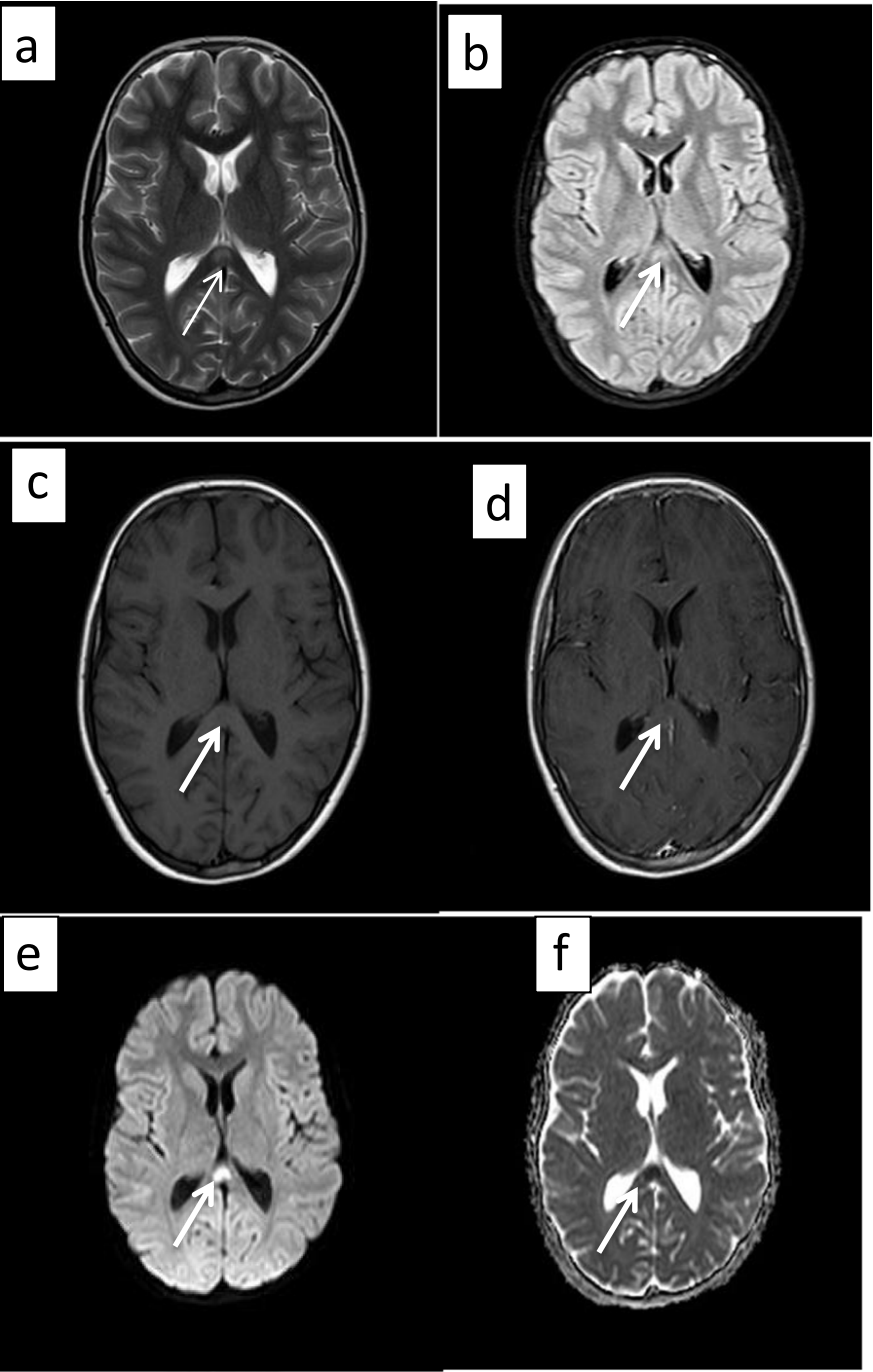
CLOCC in an 11-year-old boy with MIS-C. Brain MRI shows a lesion in the splenium of the corpus callosum (arrows) which is hypersignal on T2W (a) and FLAIR (b) sequences and was hyposignal on pre-contrast T1W sequence (c). There is no abnormal enhancement after the administration of the contrast agent (d). There is also increased signal intensity on DWI sequences at b 1000 s/mm2 (e) associated with loss of signal on the apparent diffusion coefficient map (f). These findings represent a focus of cytotoxic edema in the splenium of the corpus callosum
